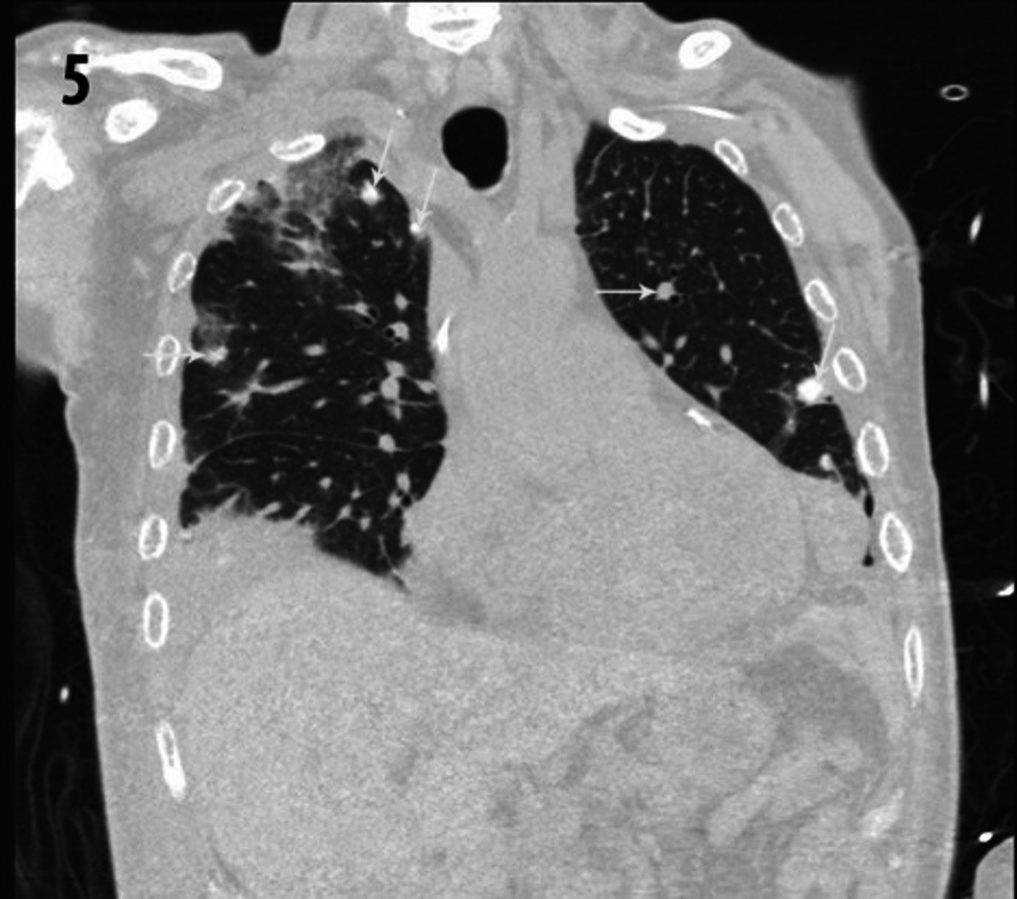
Coronal CT image of the chest using lung window levels. Multiple calcified pulmonary nodules in a random distribution (white arrows) compatible with hematogenous spread of osteosarcoma metastases. CT- Computed Tomography
